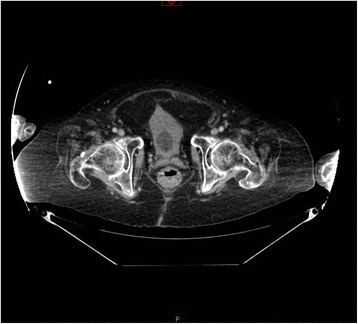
Follow-up non-contrast CT of abdomen, performed three months after cystoscopic clot evacuation: Axial section shows intact bladder outline; no perivesical haematoma.
